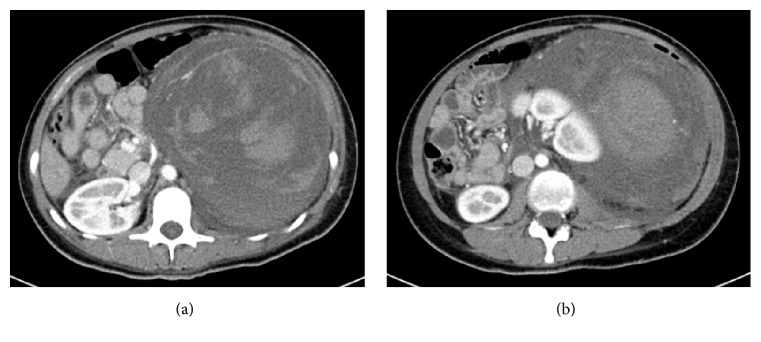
Transverse CT abdomen showing haematoma of varying ages and displaced left kidney.
